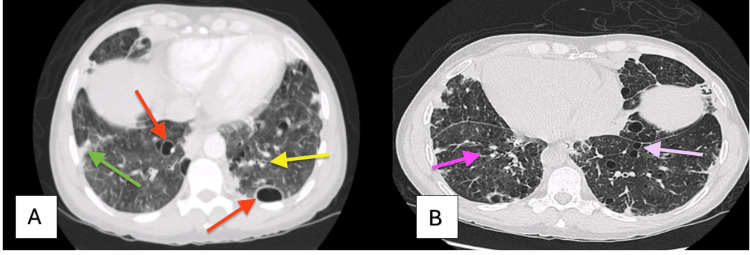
(A) CT of the chest showing diffuse cystic lesions (red arrows), bronchiectasis (yellow arrow), and ground-glass opacities (green arrow). (B) CT of the chest showing bilateral bronchiectasis (pink arrow) and multifocal cystic changes (light pink arrow).
